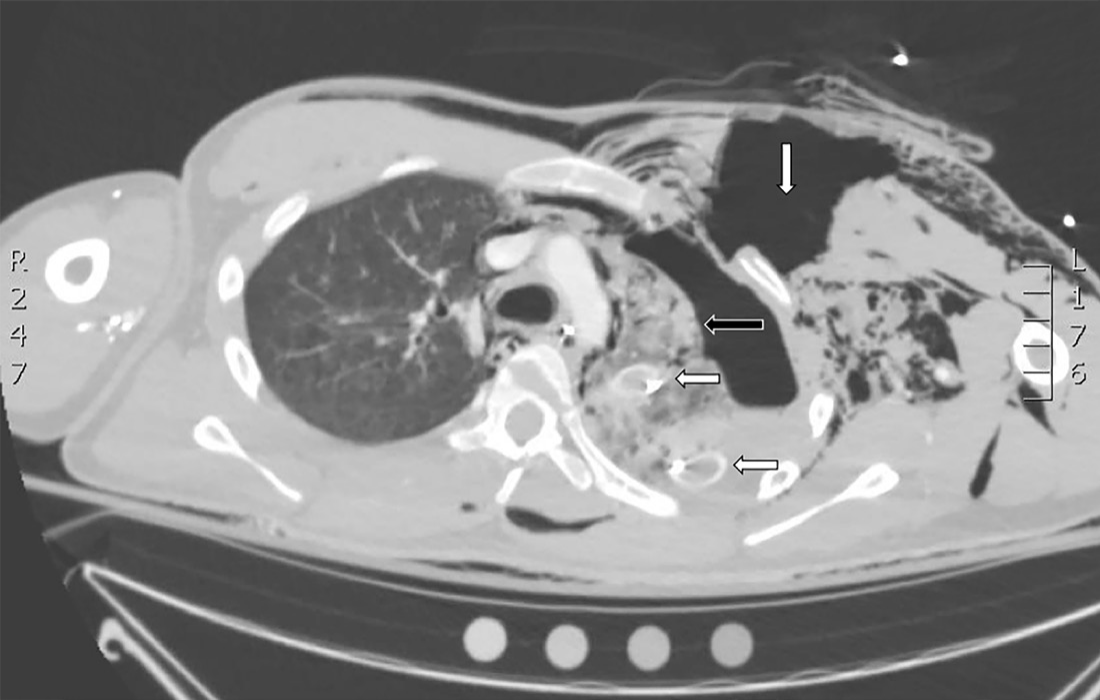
Chest computed tomography scan at the level of the aortic arch demonstrating left-sided pneumothorax (horizontal black arrow) with high-density pleural effusion compatible with hemothorax. There is a large tear of the left pectoral muscles with distraction of the muscle tissue, and a large, air-filled, 5 cm- wide defect (vertical white arrow). Two chest tubes can be seen in the left hemithorax (horizontal white arrow). A large amount of subcutaneous emphysema tracking along the chest wall can also be appreciated.
